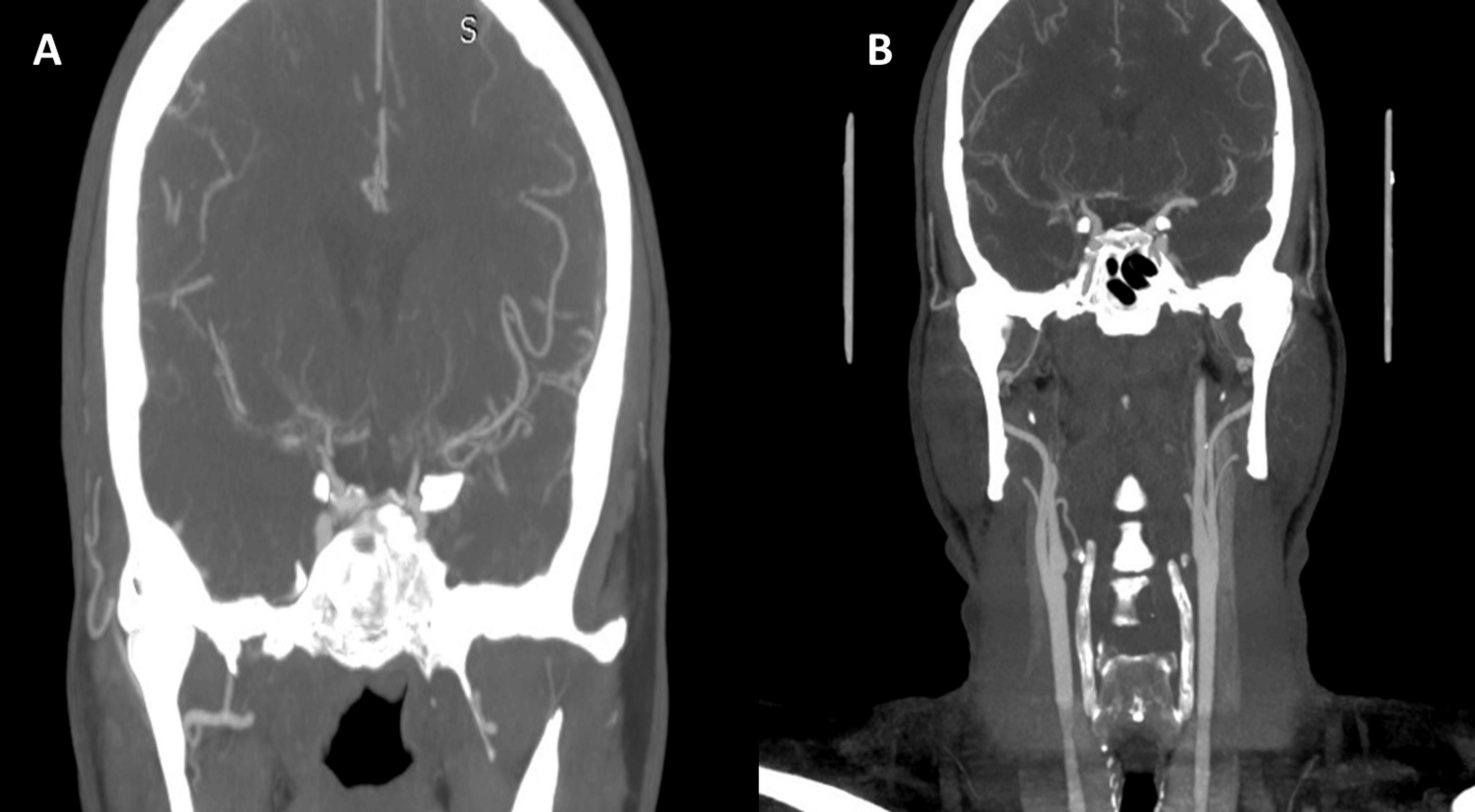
CT angiography: (A, B) irregular stenosis of the distal portion of the right ICA CT: computed tomography, ICA: internal carotid artery
